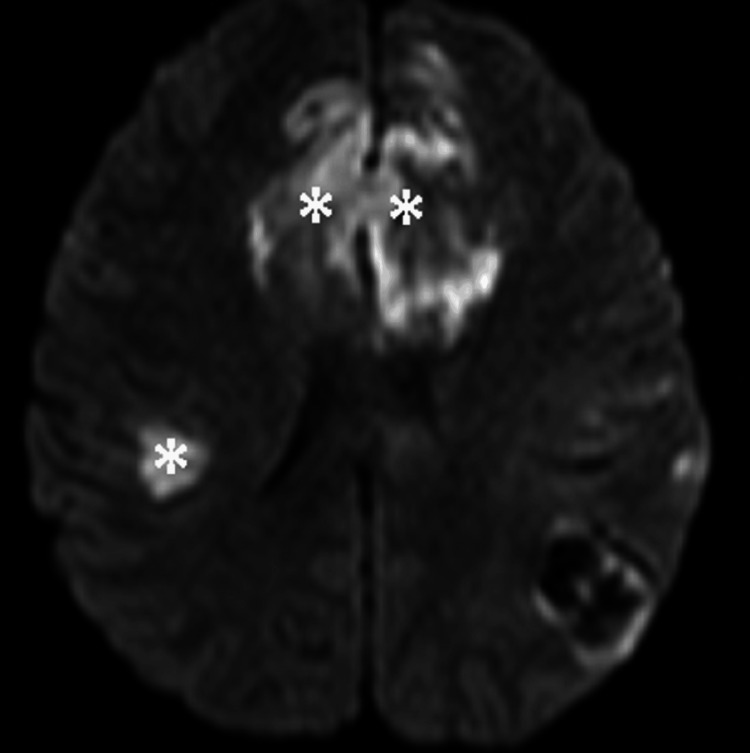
Axial non-enhanced MRI of the brain. Diffusion-weighted image shows areas of high signal and low ADC (not shown) involving the bi-frontal and right parietal lobes (asterisks) consistent with infarcts MRI: magnetic resonance imaging, ADC: apparent diffusion coefficient
